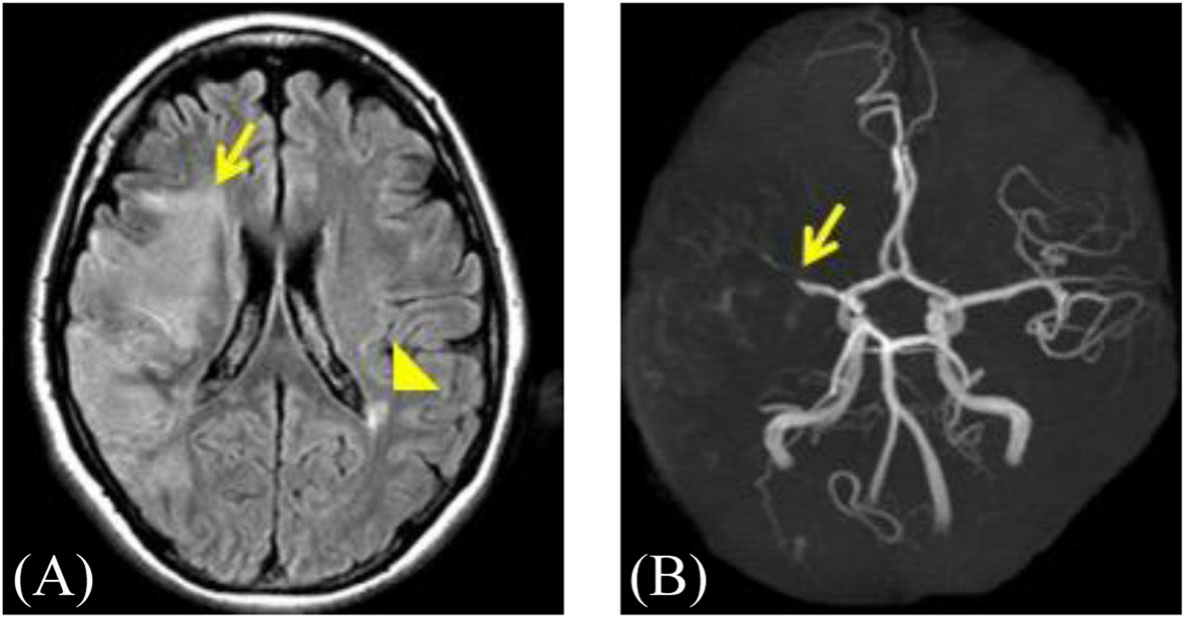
a Magnetic resonance imaging of the brain. Diffusion-weighted image demonstrating massive right cerebral infarction in the cerebral cortex (arrow) and another infarction (arrowhead) in the left hemisphere. b Magnetic resonance (MR) angiography of cerebral arteries. MR angiography demonstrating total occlusion of the M1 segment in right middle cerebral artery (arrow)
